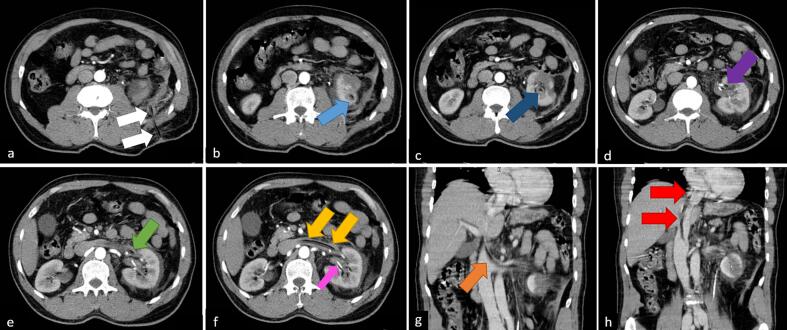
Abdominopelvic CT scan with IV contrast. a. The nephrostomy tube from skin to kidney (white arrow). b. The tube entering kidney from access tract (light blue arrow). c. The tube in renal pelvis (dark blue arrow). d, e. The tube entering renal vein (purple and green arrow). f. The tube in the left renal vein (yellow arrow), Part of double J stent in kidney (pink arrow). g. The tube traversing left renal vein and IVC (orange arrow). h. The tube entering right atrium (red arrow). (For interpretation of the references to color in this figure legend, the reader is referred to the web version of this article.)
